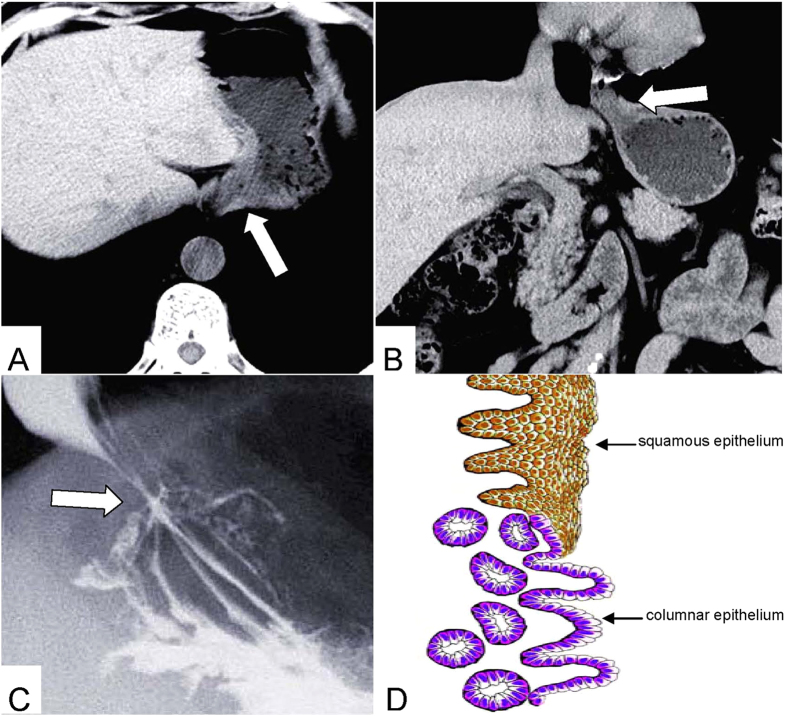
CT axial view image (A) coronal view image (B) Gas-barium double-contrast X-ray image (C) and sketch map (D) of the esophagogastric junction: (A,B) CT demonstrates the esophagogastric junction and the relation with adjacent tissues(the empty arrow). (C) Mucosa shows stellate high-density shadow (the empty arrow).
